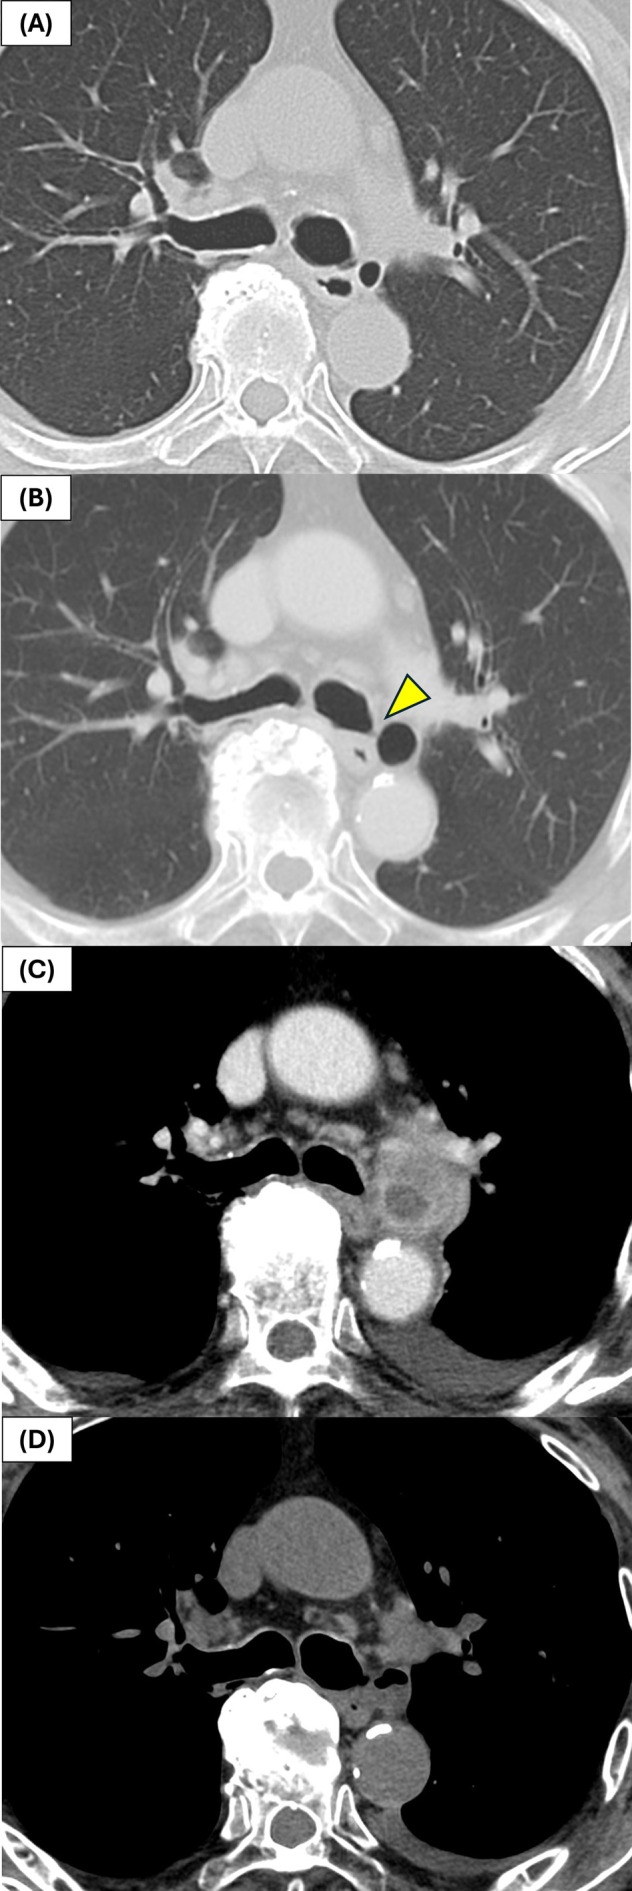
Serial chest computed tomography (CT) images demonstrating the progression of bronchial diverticulum (BD). (A) In 2013, an 8‐mm BD was observed adjacent to the left main bronchus. (B) In 2020, the BD had enlarged to 14 mm, with clear evidence of communication with the bronchial lumen (yellow arrow). (C) In 2024, the diverticulum had further increased in size to 18 mm, with internal fluid accumulation and increased attenuation of the surrounding adipose tissue, indicating inflammation. (D) One‐month post‐treatment, the size of the diverticulum remained unchanged, with a mixture of fluid and gas inside, but the attenuation of the surrounding adipose tissue had returned to normal.
